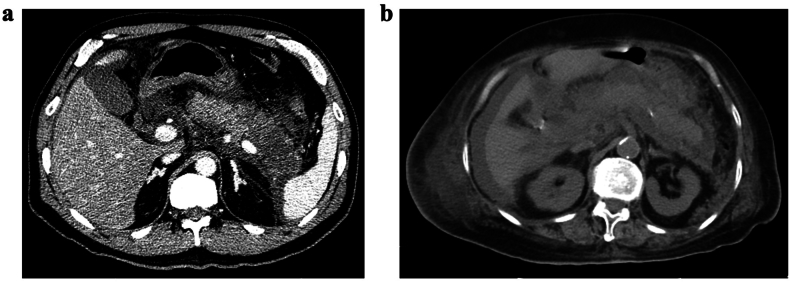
Abdominal CT showed SAP before the starting of WMT (a: Case 1; b: Case 2).
